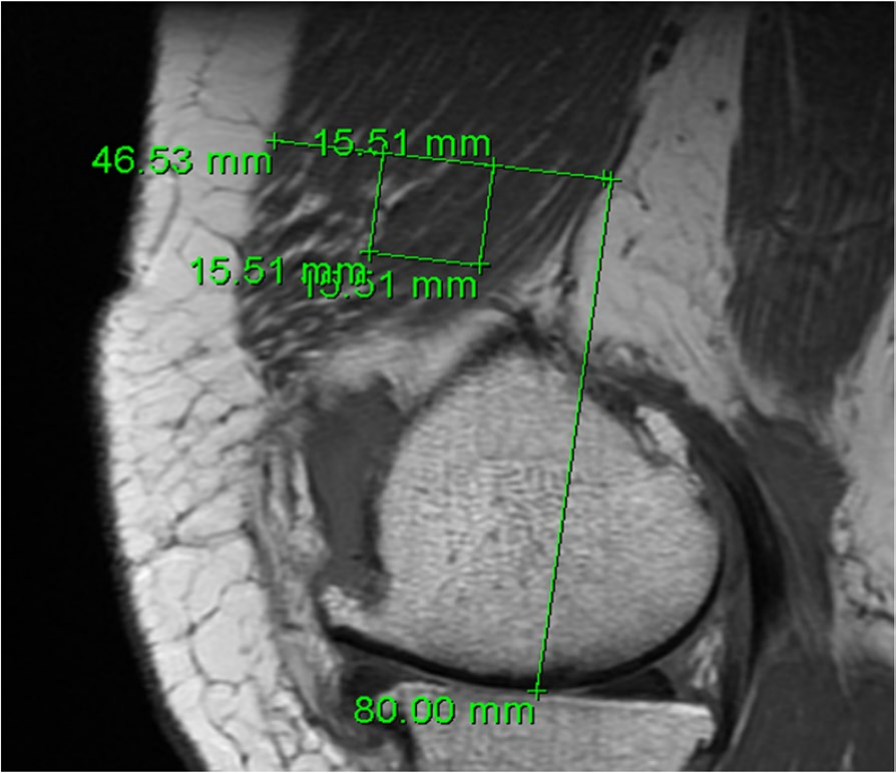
Location of the region of interest (ROI) in the vastus medialis muscle in a sagittal T1-weighted magnetic resonance image (3 T). The ROI was set 8 cm above the joint space, in the middle of the vastus medialis muscle
